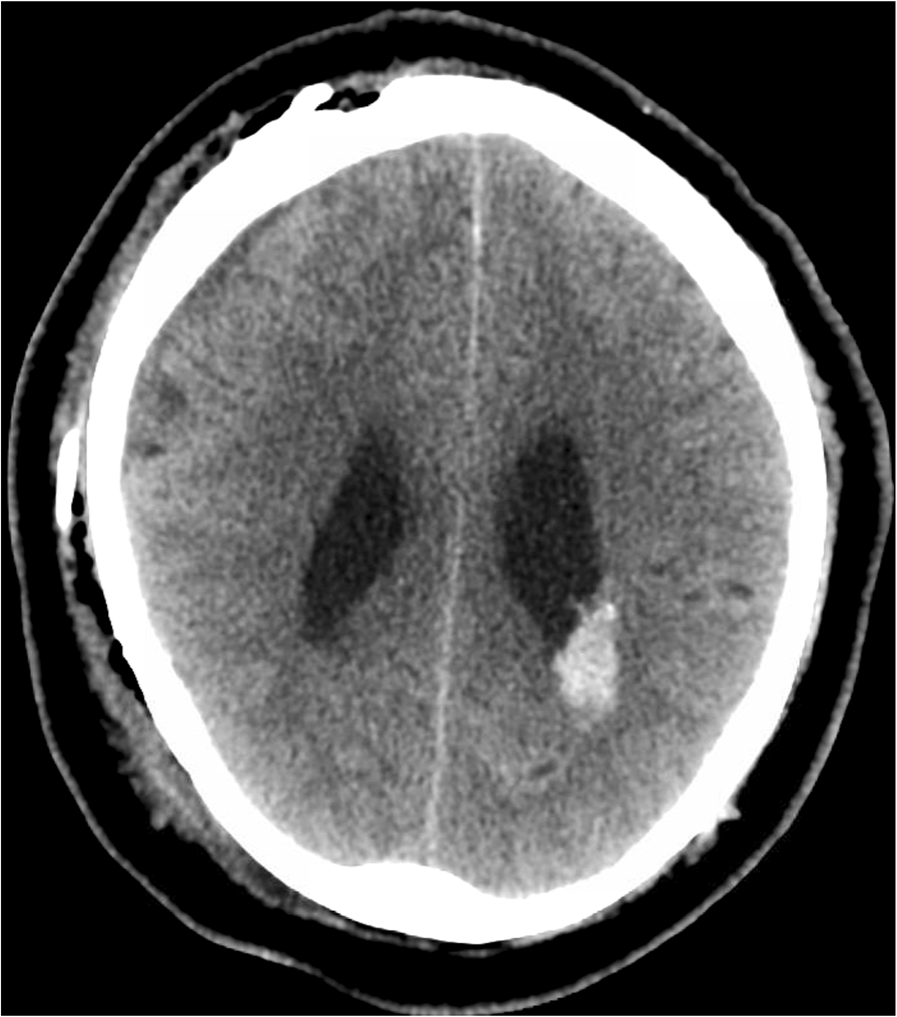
CT head post op day 1 after ventriculoperitoneal shunt, showing interval hemorrhage of cavernous malformation
